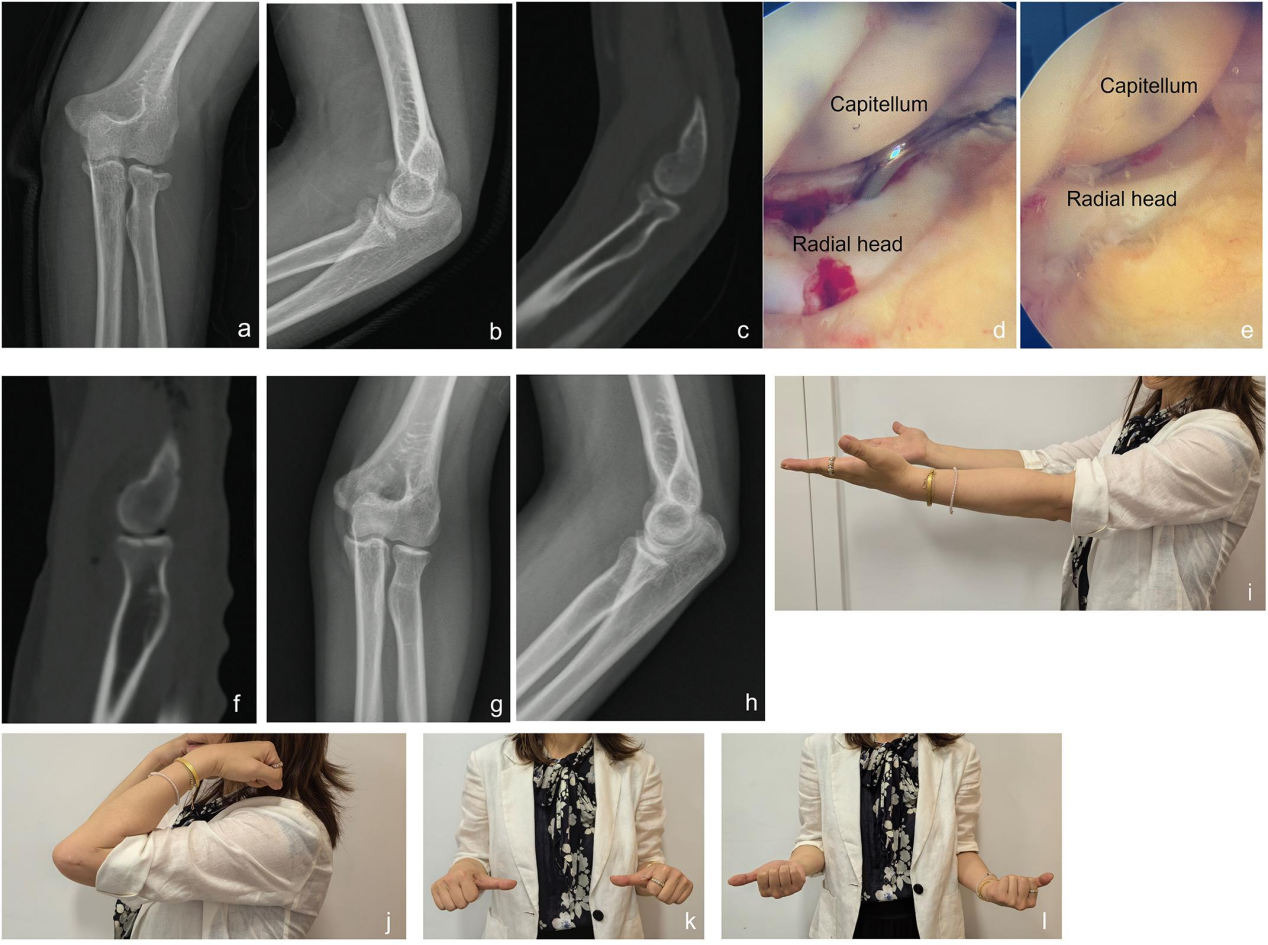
A case example for a patient treated with arthroscopic reduction with non-fixation. A 38-year-old woman (Patient 8) experienced a left radial head fracture due to an accidental fall. (a,b) X-ray images after injury; (c) CT-scan image after injury; (d) intraoperative arthroscopic image showed fragment of radial head; (e): intraoperative arthroscopic image showed that the fragment was stable after reduction; (f) postoperative CT-scan image; (g,h) x-ray images at 6 months follow-up; (i–l) range of motion at 6 months follow-up.
